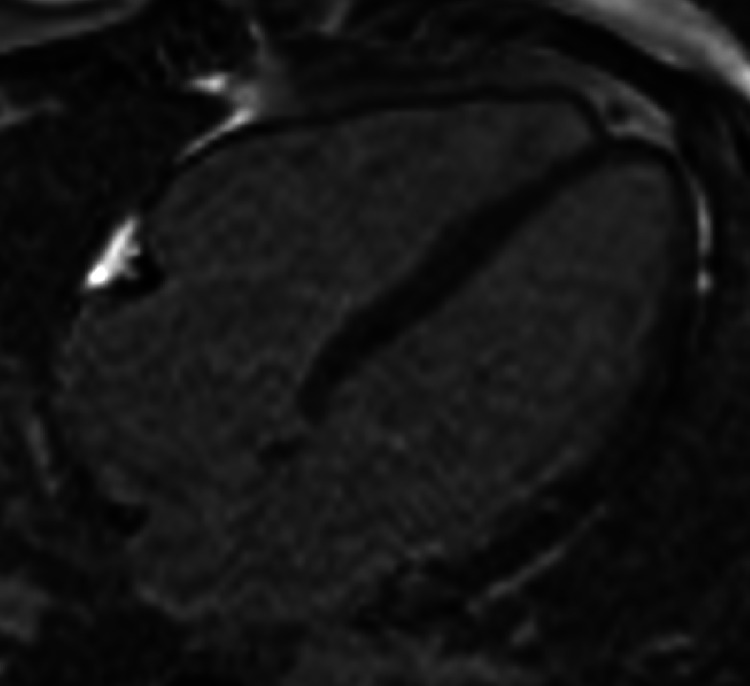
1.5 T MRI postcontrast T1 weighted sequence showing the absence of subendocardial late gadolinium enhancement and thus no signs of myocardial infarcts.
